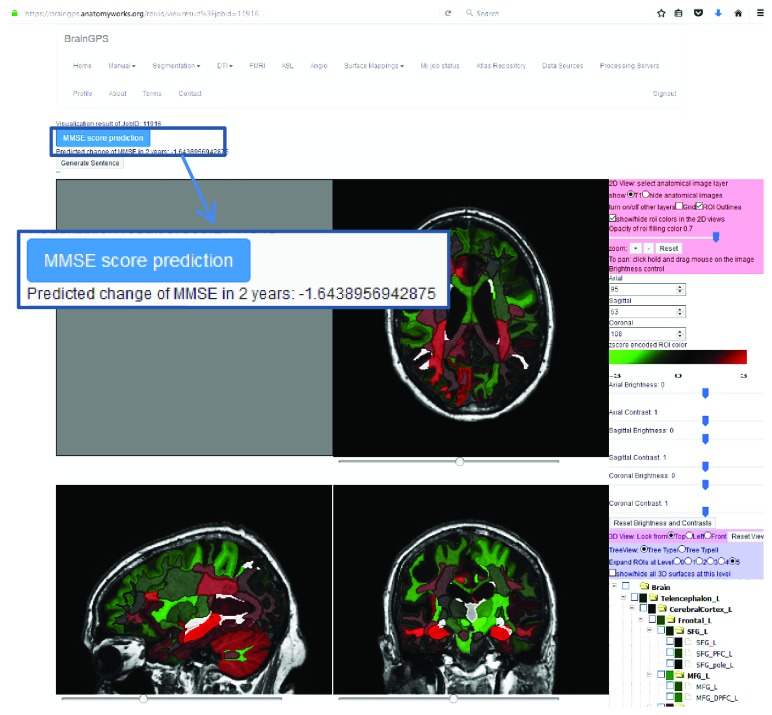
Screenshot of the MMSE score prediction function implemented in the BrainGPS module of the MRICloud. The module allows users to submit their own high-resolution, 3D, T1-weighted images with the age and MMSE score at scan. The module provides a color-coded z-score map of the local volume (lower left) as well as the predicted ΔMMSE (magnified view in the blue rectangle).
